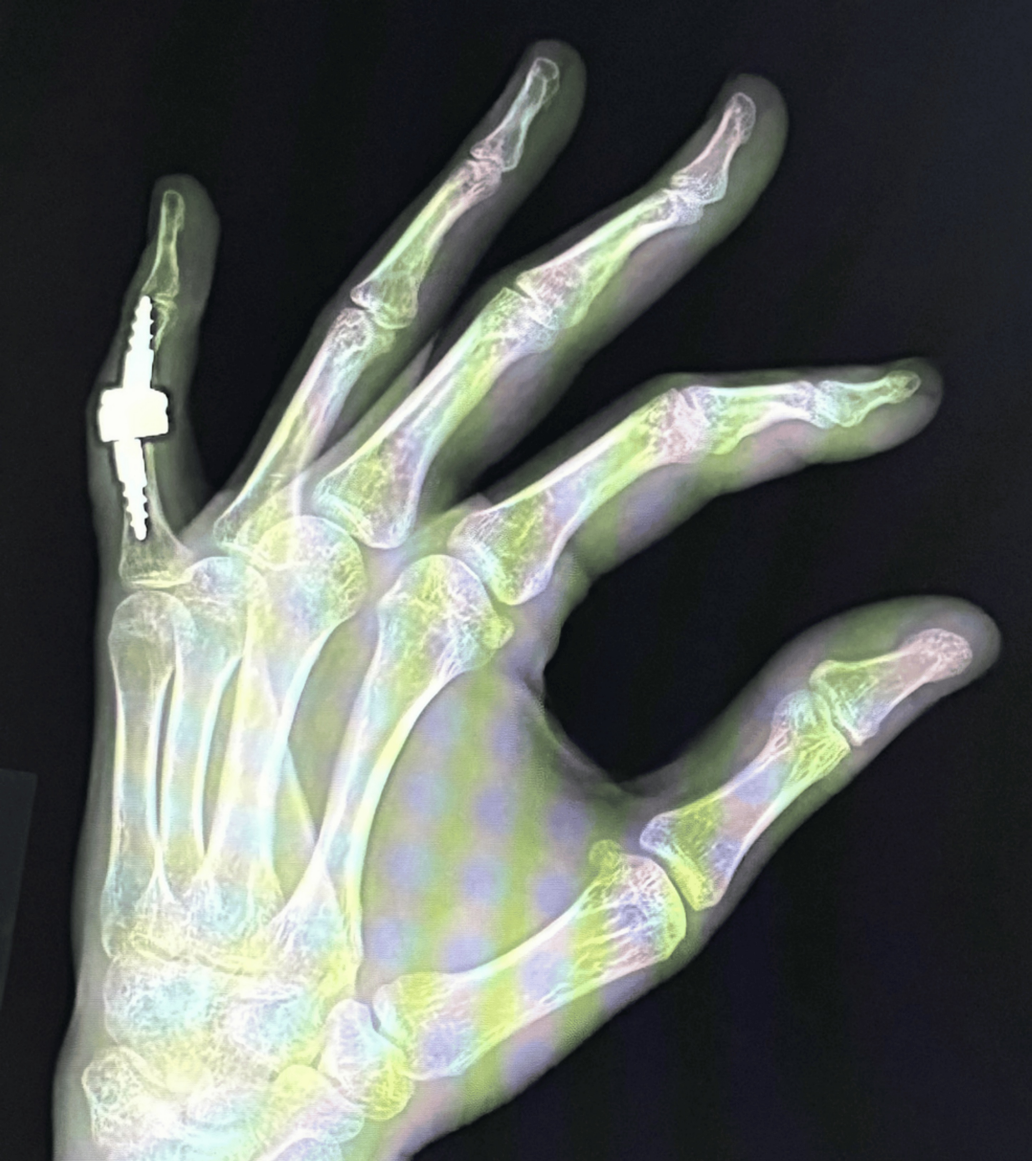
Control X-ray eight weeks after implantation of the 3S ORTHO prosthesis in Robert's projection
